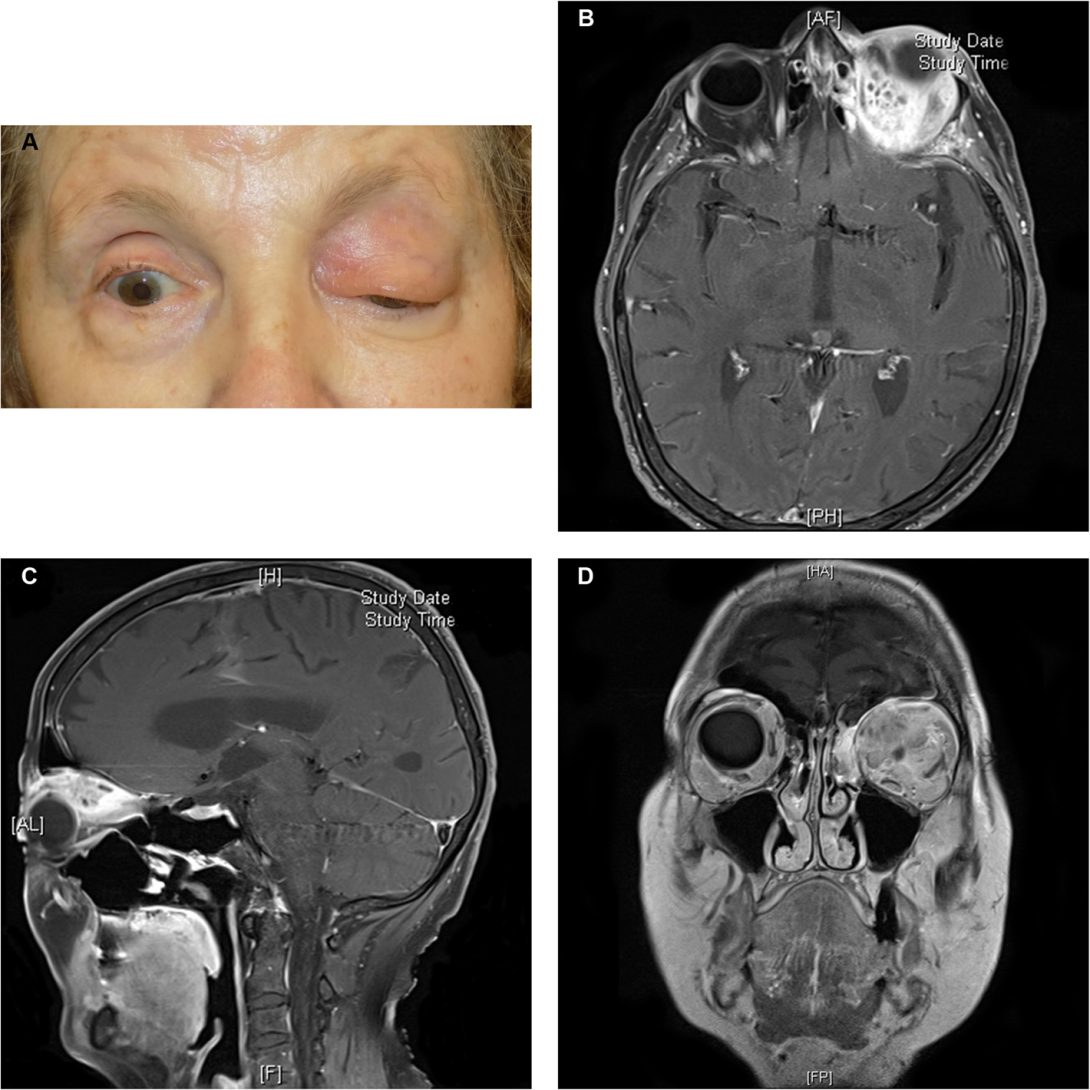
Clinical and radiologic presentation before therapy. a shows an en-face photograph of the patient 2 months after the beginning of symptoms. Axial (b) and sagittal (c) fat-suppressed T1-weighted and coronal (d) T1-weighted (without fat suppression) MR images showing the inhomogeneous mass in the upper nasal part of the left orbit
